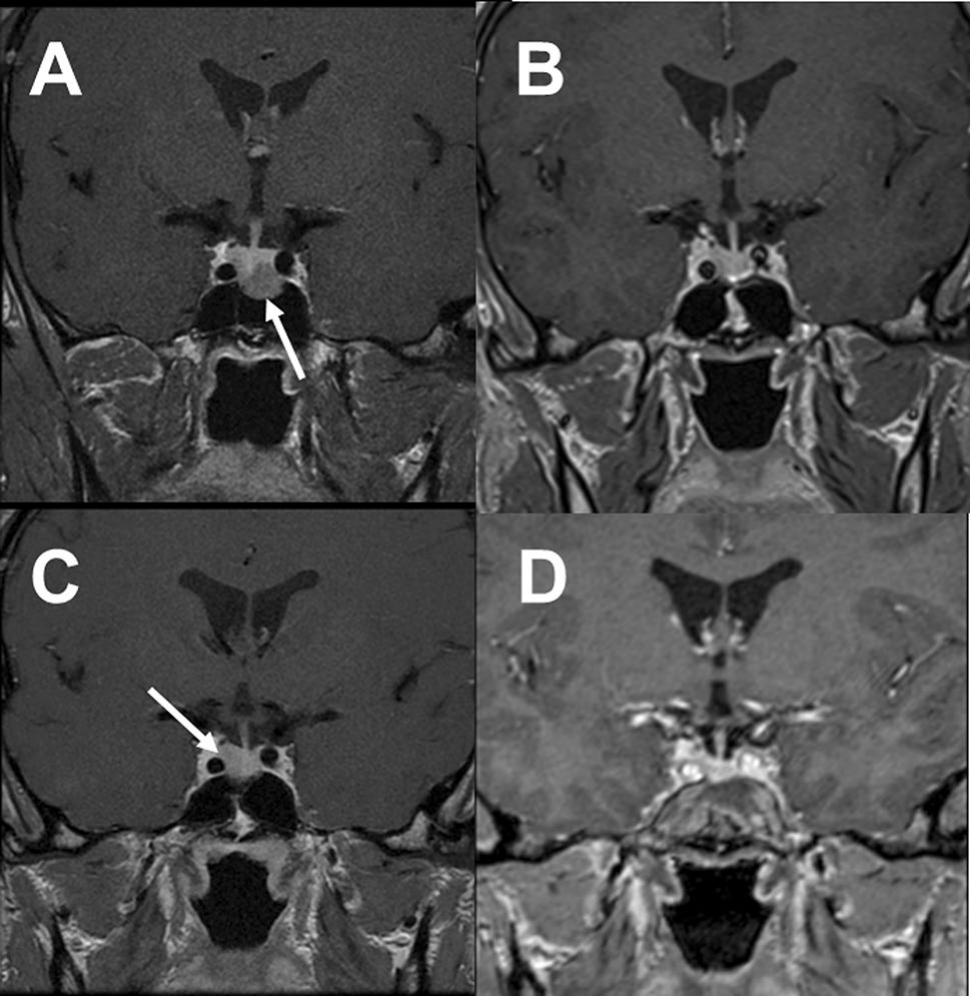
Case no. 12: a arrow indicates somatotroph pituitary macroadenoma removed with its pseudocapsule during first transsphenoidal operation (year 2008), b postoperative MR image (after first operation), remission of the acromegaly was achieved, c arrow indicates a new pituitary adenoma in the right lateral wing of the pituitary gland (year 2011), d postoperative MR image (after second operation)
